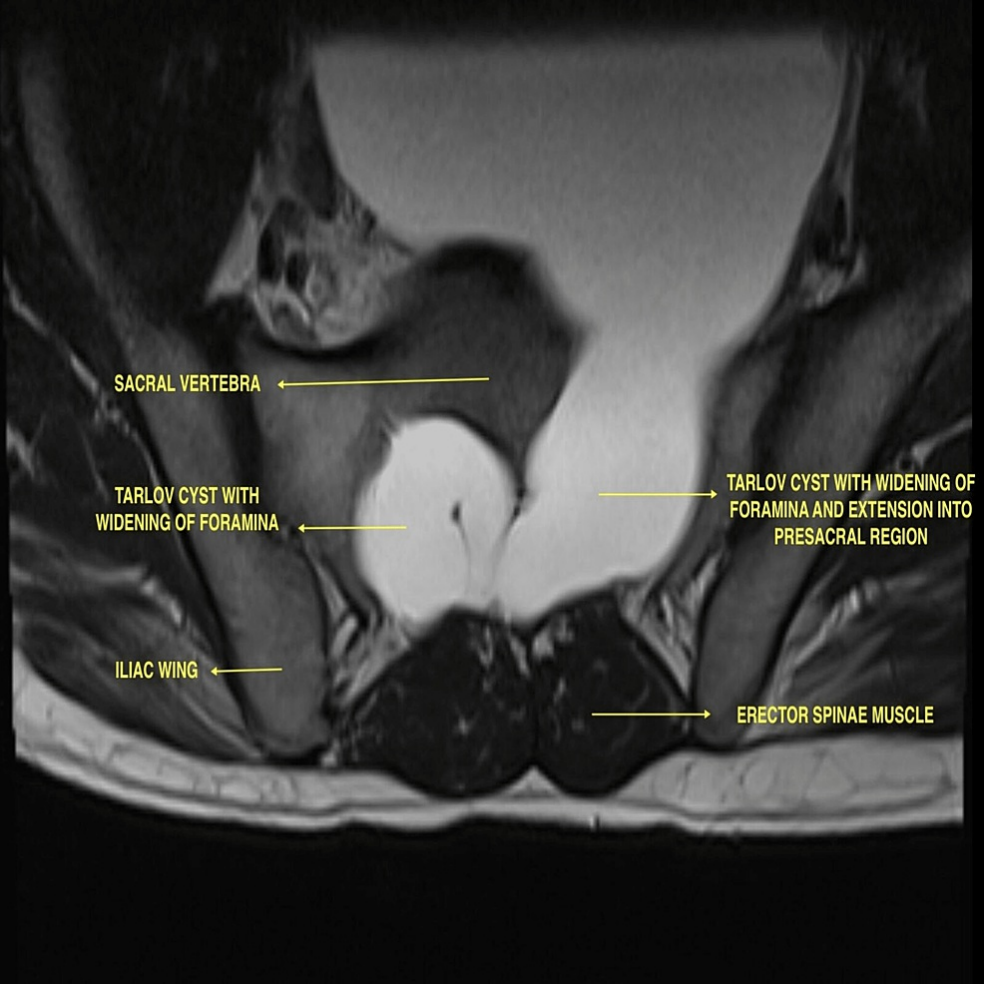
T2 MRI transverse axial image depicting giant Tarlov cysts
